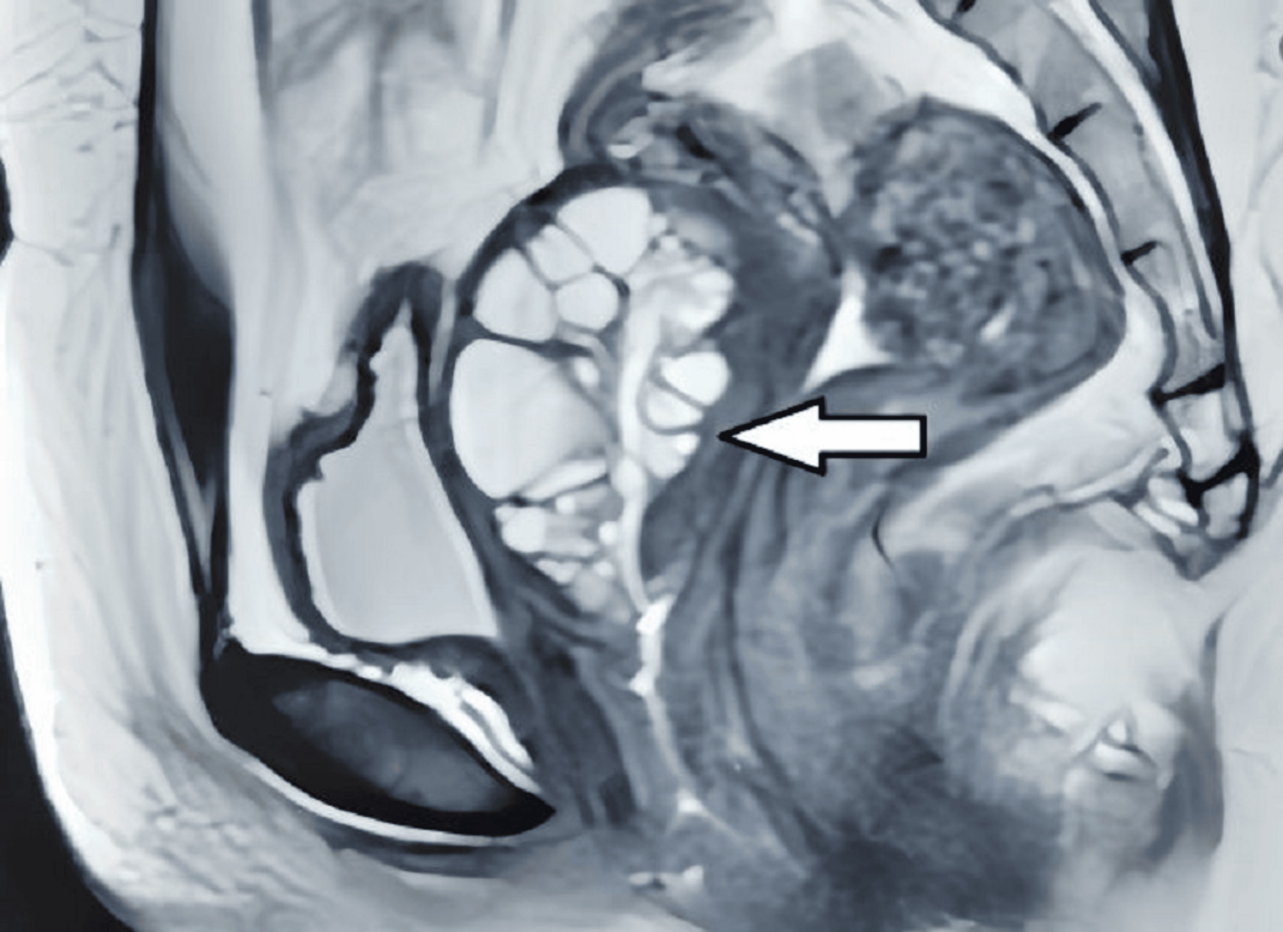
T2-weighted MRI showing the sagittal section of the pelvis. A hyperintense septated cystic lesion involving the cervix is noted.
